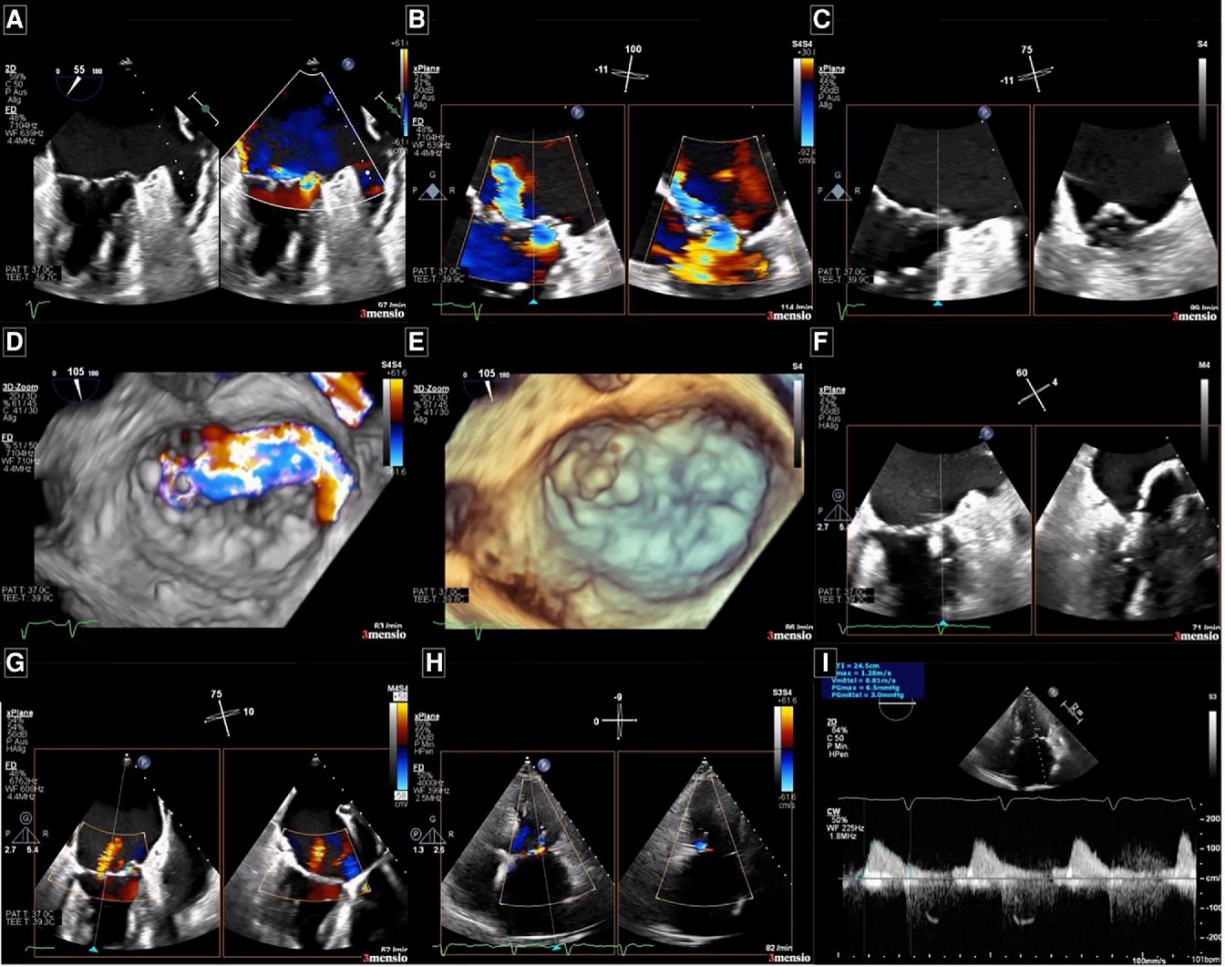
Diagnostic and intraprocedural echocardiographic imaging of the mitral valve before and after the first mitral transcatheter edge-to-edge repair procedure. (*A*) Mitral commissural view with simultaneous colour Doppler showing an eccentric regurgitant jet originating from the lateral commissure. (*B*) Zoomed biplane view through the lateral segments (A1/P1) of the mitral valve leaflets with colour Doppler. (*C*) Same biplane view without colour Doppler revealing a flail posterior mitral valve leaflet, the main pathology causing severe mitral regurgitation. (*D*) 3D en face view of the mitral valve during systole, with the aortic valve positioned at 12 o'clock, showing the eccentric jet originating from the A1/P1 segment. (*E*) 3D transoesophageal echocardiography image highlighting the flail posterior mitral valve leaflet responsible for the severe mitral regurgitation prior to MitraClip (Abbott®) placement. (*F*) Intraprocedural biplane imaging during device positioning targeting the lateral commissure. (*G*) Marked reduction in mitral regurgitation severity after initial clip deployment. (*H*) Post-procedural transthoracic echocardiography showing trace residual mitral regurgitation. (*I*) A mean mitral valve gradient of 3.0 mmHg was measured following the first clip implantation.
